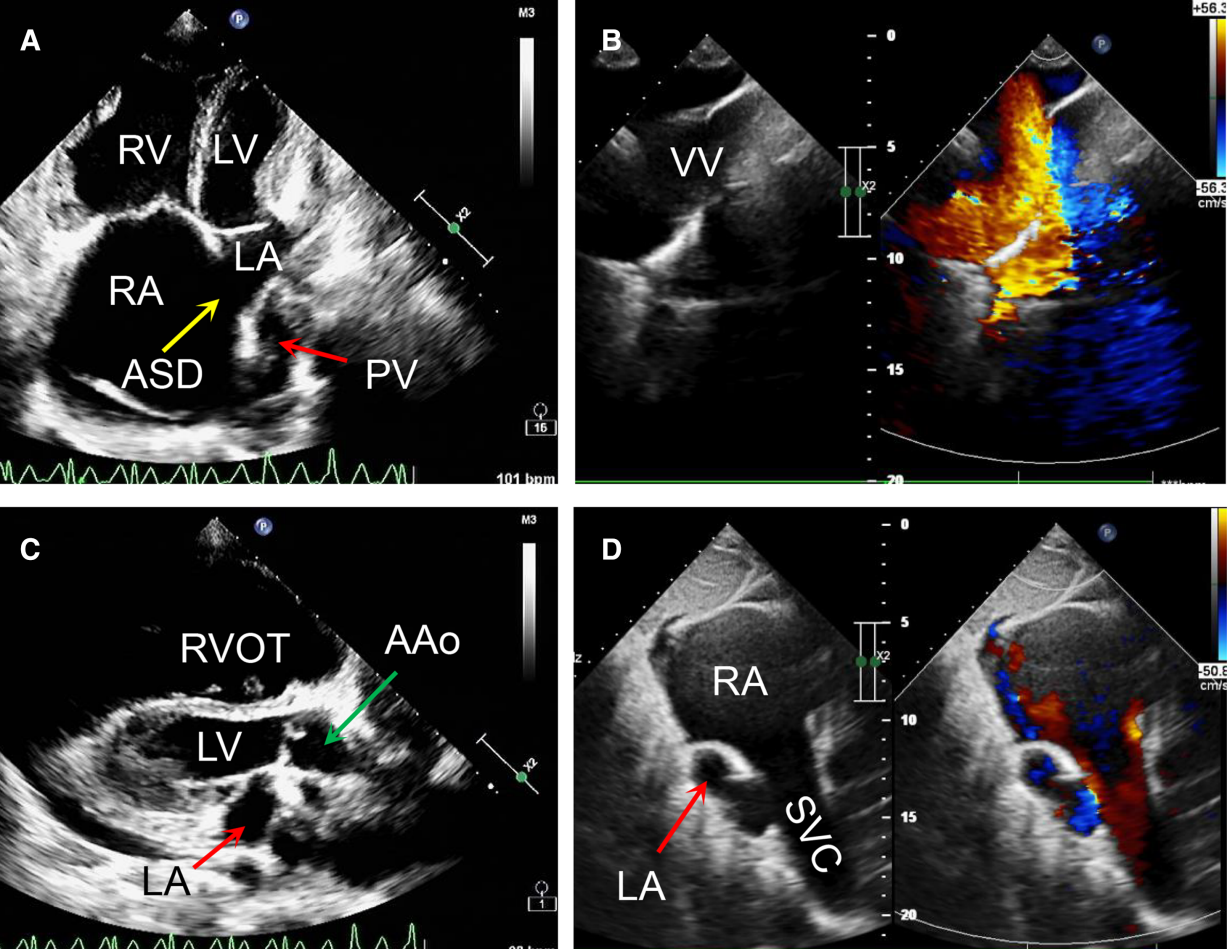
Transthoracic echocardiography preoperatively revealed that a right to left atrial septal shunt was detected with the diameter of around 40 mm (A,B); the ratio of ascending aorta to the pulmonary trunk was 24:32 (C); the left and right pulmonary veins returned to a vertical vein then innominate vein and the superior vena cava. (D) AAo, ascending aorta; ASD, atrial septal defect; LA, left atrium; LV, left ventricle; PV, pumonary vein; RA, right atrium; RV, right ventricle; RVOT, right ventriclar outflowtract; SVC, superior vena cava; VV, vertical vein.
